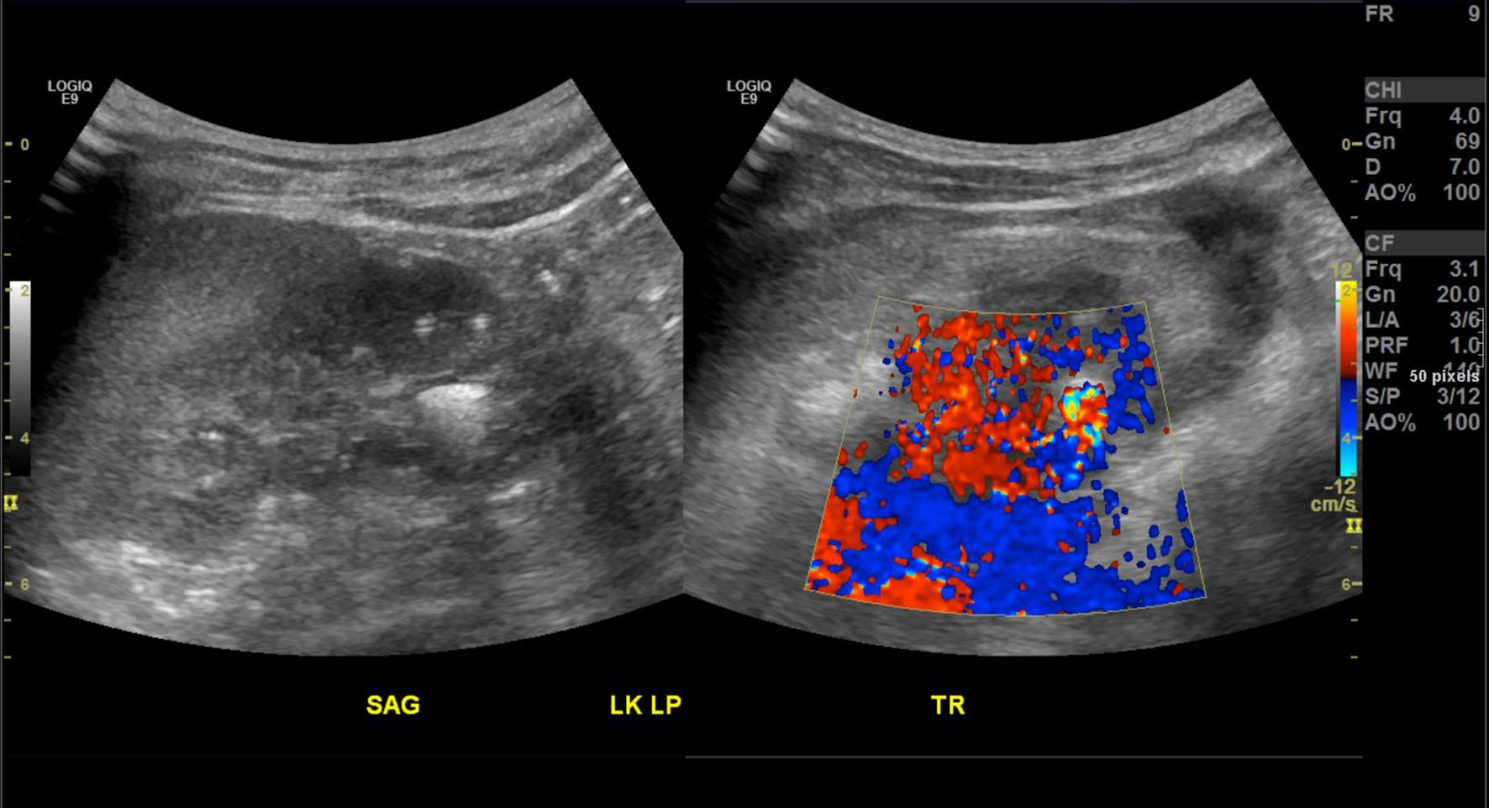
In vivo ultrasound of xanthine urinary stone: stone demonstrates posterior twinkle artifact on color Doppler imaging
